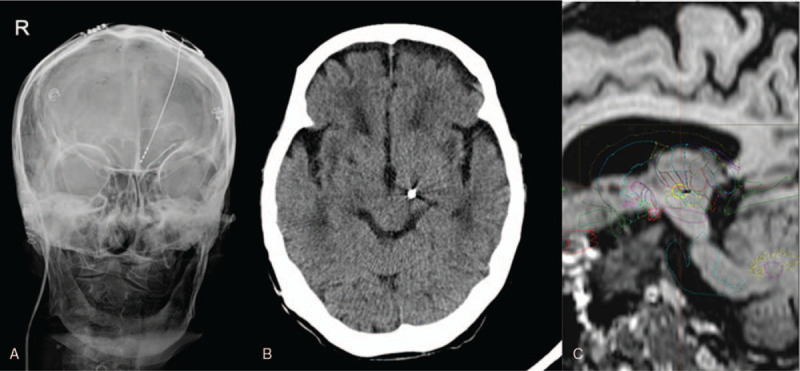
Post. DBS Skull X-ray (A) and axial CT (B) placement of the electrode in the left ventralis oralis anterior nucleus. A sagittal MRI image (C) superimposed on the Schaltenbrand–Wahren atlas using planning software.
